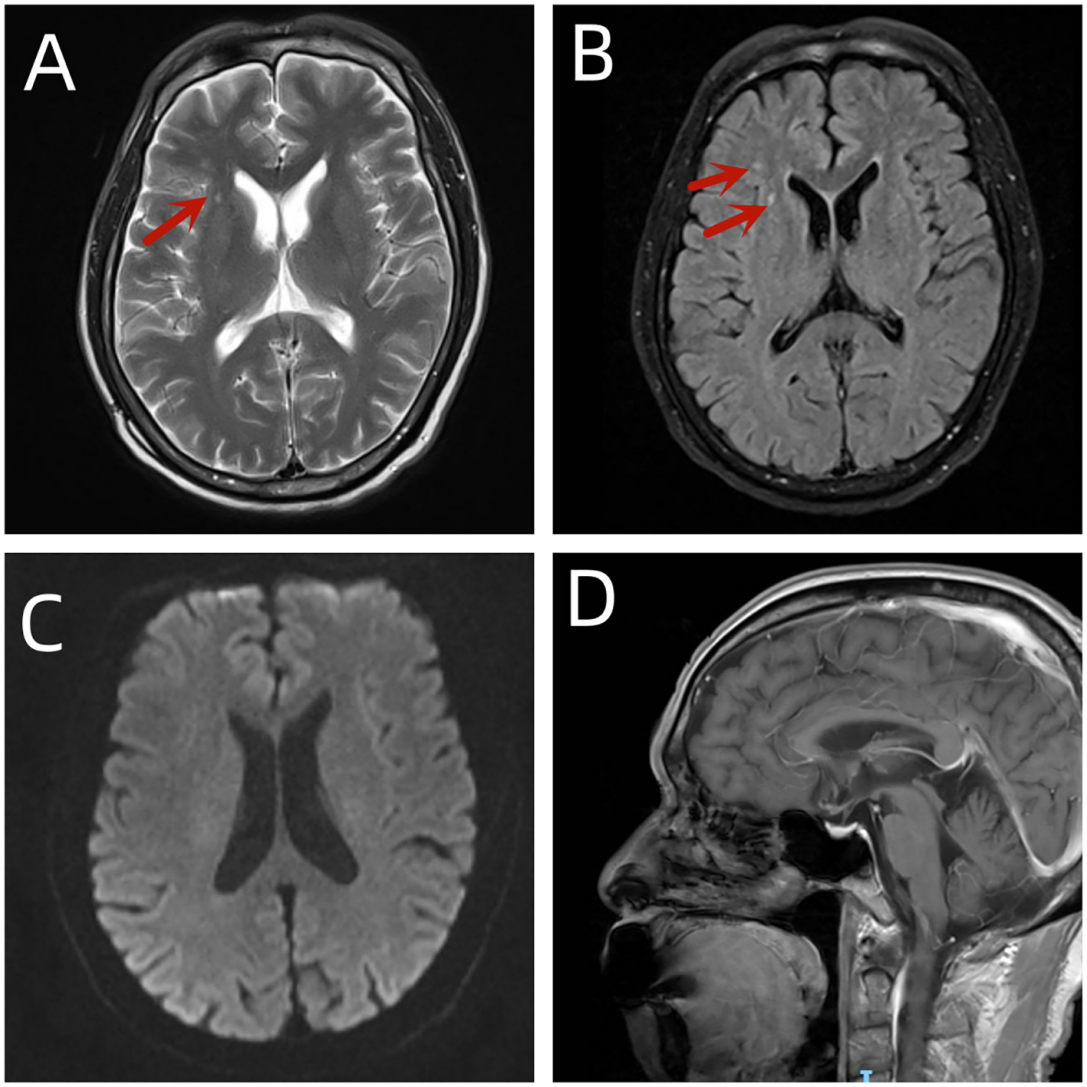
Four MRI brain scans labeled (A–D). (A) Axial view showing a bright area indicated by an arrow. (B) Axial view with two arrows highlighting affected areas. (C) Axial view without annotations, showing symmetrical brain structures. (D) Sagittal view displaying the brain's side profile, detailing structures like the cerebellum and brainstem.
